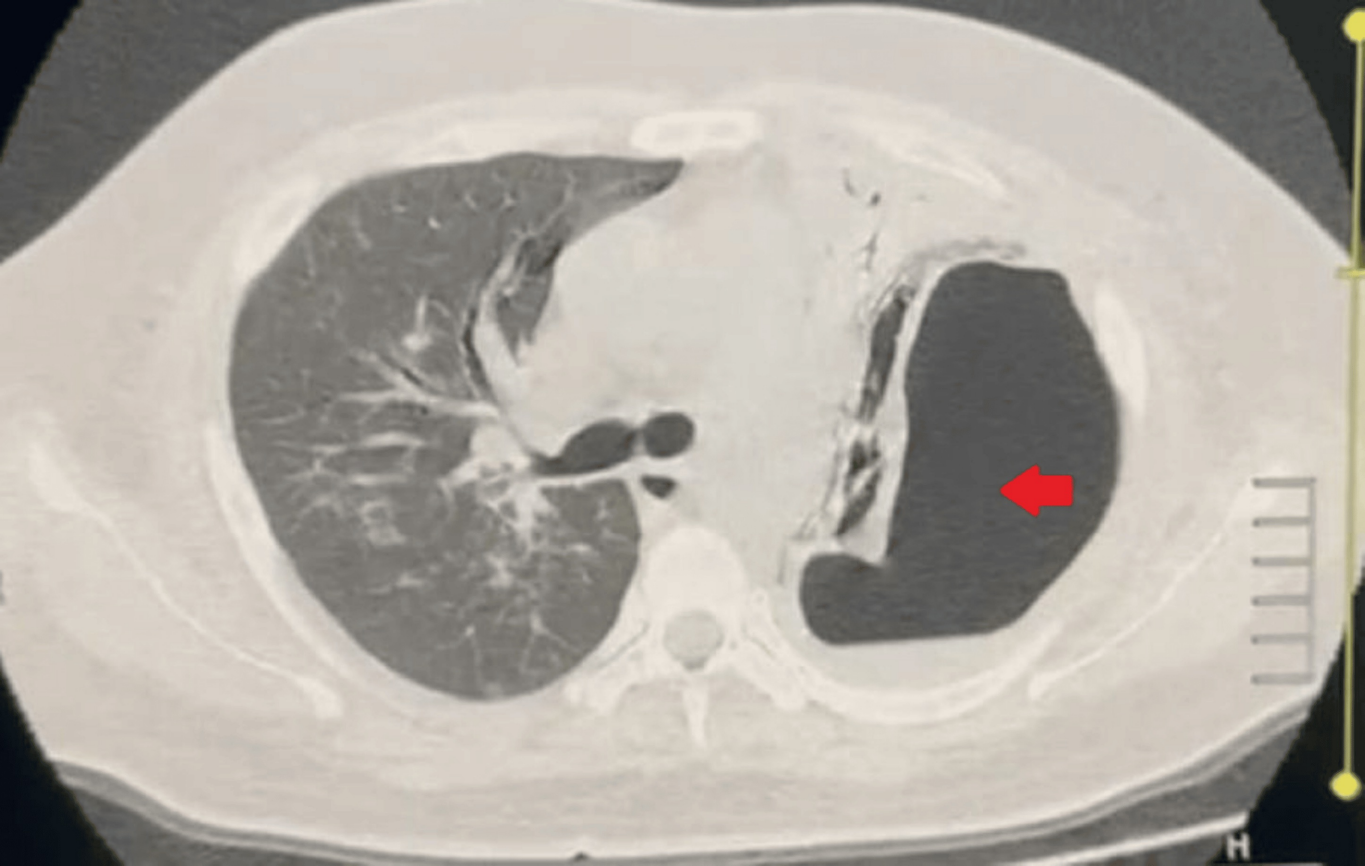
HRCT of the chest: axial view (patient 2) HRCT of the chest demonstrating an encysted left-sided hydropneumothorax measuring 10 cm × 7.8 cm × 12 cm with collapsed left lung. Peribronchovascular patchy consolidation with ground glassing noted in the right lung with upper lobe predominance. The red arrow indicates the left-sided pneumothorax HRCT: high-resolution computed tomography
